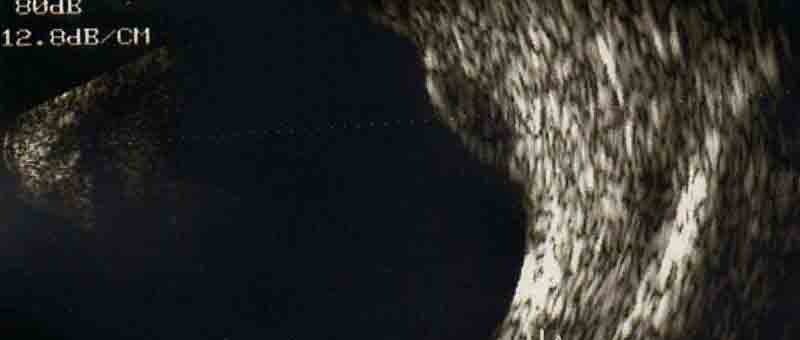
B-scan ultrasound of the involved eye. B-scan ultrasound showing flat-surfaced, elevated choroidal lesion with moderate internal reflectivity.
